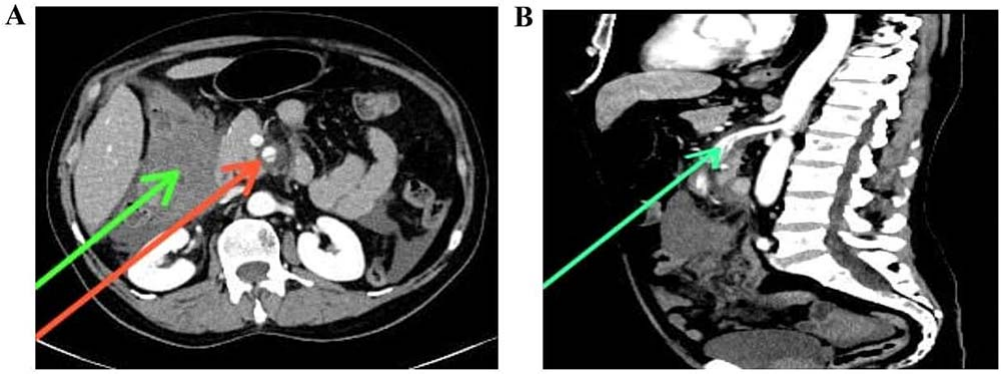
(A) CT aortogram (axial view at the level of the kidneys) showing an intimal flap dissecting the SMA (red arrow) and hemoperitoneum in the right hypochondriac region (green arrow). (B) CT aortogram (sagittal view, arterial phase) demonstrating a focal intimal flap dissecting the SMA.
